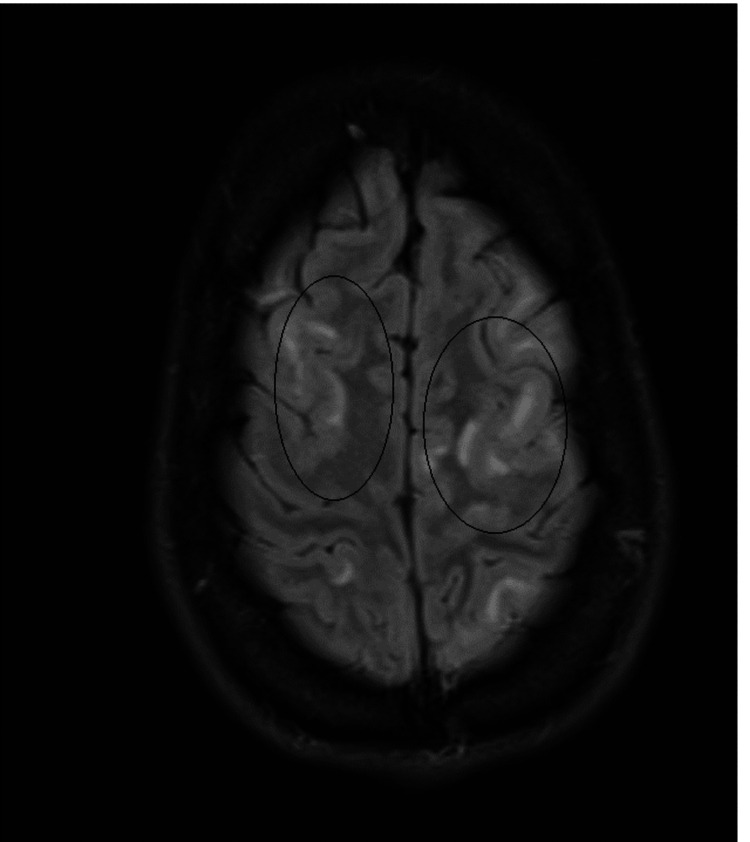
T2-FLAIR sequence axial section at a level near the vertex. This image shows widespread juxtacortical and cortical signal changes. At other levels (not shown in this image), there was also partial effacement of sulcal spaces, particularly in the left temporal region FLAIR: fluid-attenuated inversion recovery
